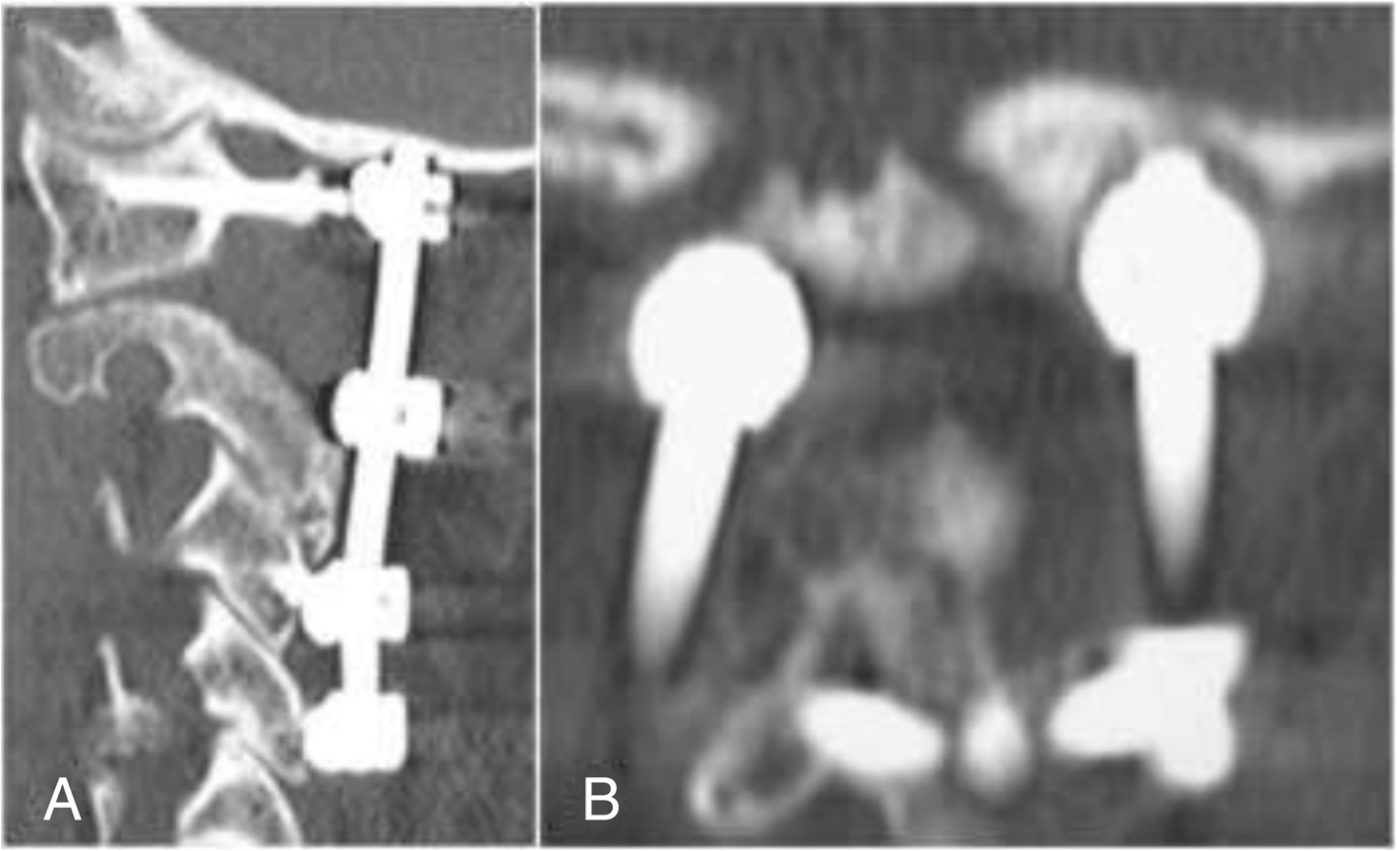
MPR-CT images of a 61-year-old man obtained at 6 months following C1-C4 fusion. A. The sagittal image shows that the tip of the rod above the lateral mass screw protrudes into the occipital bone. B. The coronal image shows that the occipital bone is eroded by the tip of the rod on the left.
